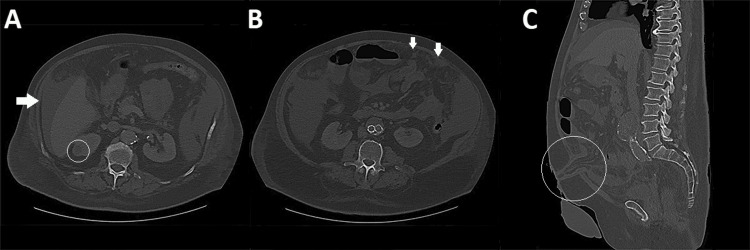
CT scan a month after surgery showing: (A) new large volume ascites (white arrow) and a new right kidney lesion measuring 2.5 cm (white circle); (B) worsening peritoneal implants (white arrows); and (C) an ileostomy (white circle).
